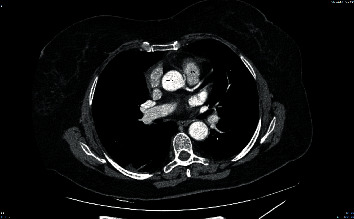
CT angiography of the aorta revealing an anomalous origin of the RCA (depicted with an “∗”). The RCA exhibits an interarterial course between the aorta and the pulmonary artery (PA).
